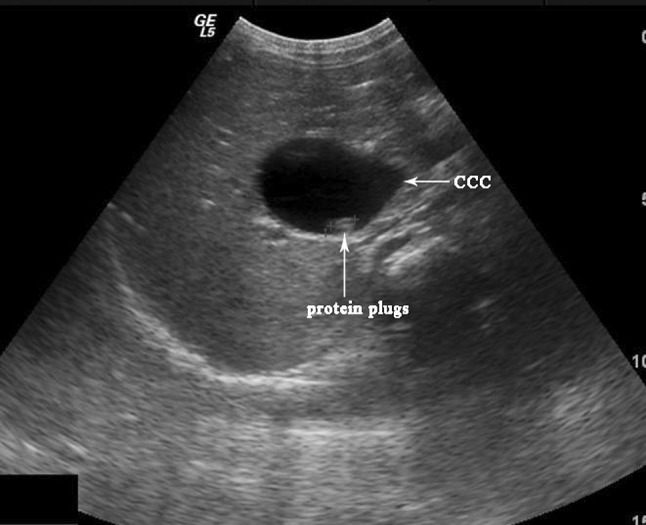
Transverse ultrasonography shows the common duct dilated with protein plugs
